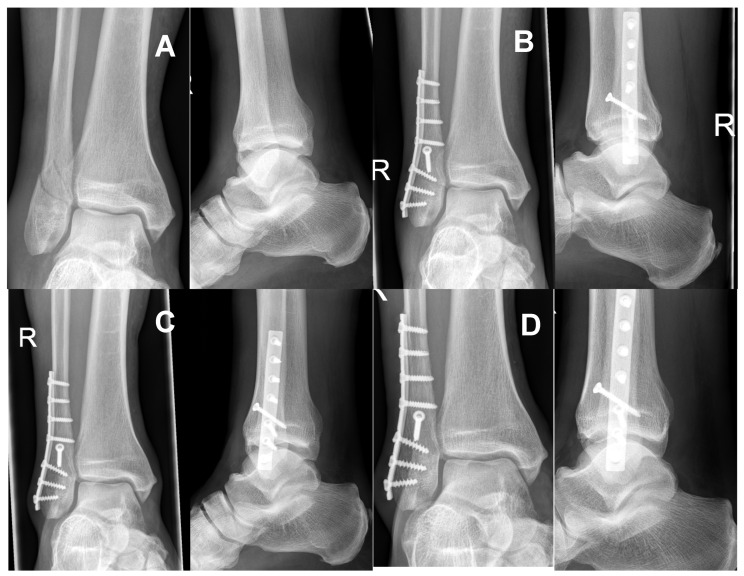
(A) shows the preoperative radiograph of a 72-year-old male patient with an AO type 44B1.1 fracture. The patient was treated with the DePuy Synthes^®^ one-third semi tubular plate system. Figure (B) presents the postoperative results after 6 and Figure (C) after 12 weeks. In addition, the 14 months postoperative control (D) shows good fracture healing without any secondary complications.
